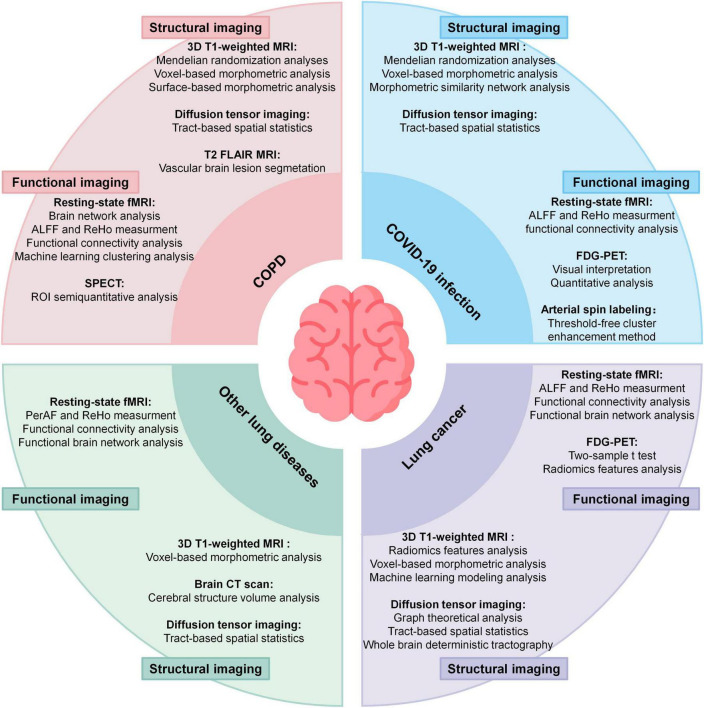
Summary of neuroimaging methods applied in 4 kind of lung disease and corresponding analyses methods. ALFF, amplitude of low-frequency fluctuations; ReHo, regional homogeneity; PerAF, percent amplitude of fluctuation; SPECT, single-photon emission computed tomography. ^18^F-FDG-PET, ^18^F-FDG positron emission tomograph.
